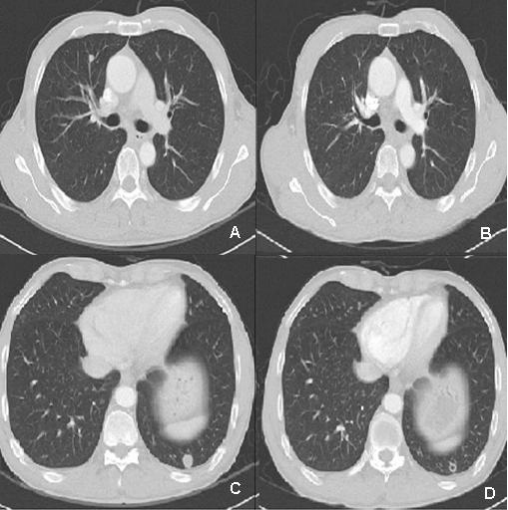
A, B: Chest CT demonstrating hematogenous metastastatic nodule in RML (arrow, A) disappeared after 4^th ^FOLFOX4 cycles (B). C, D: Chest CT demonstrating hematogenous metastastatic nodule in LLL (arrow, C) that formed fibrotic cavity after 4^th ^FOLFOX4 cycles (D).
